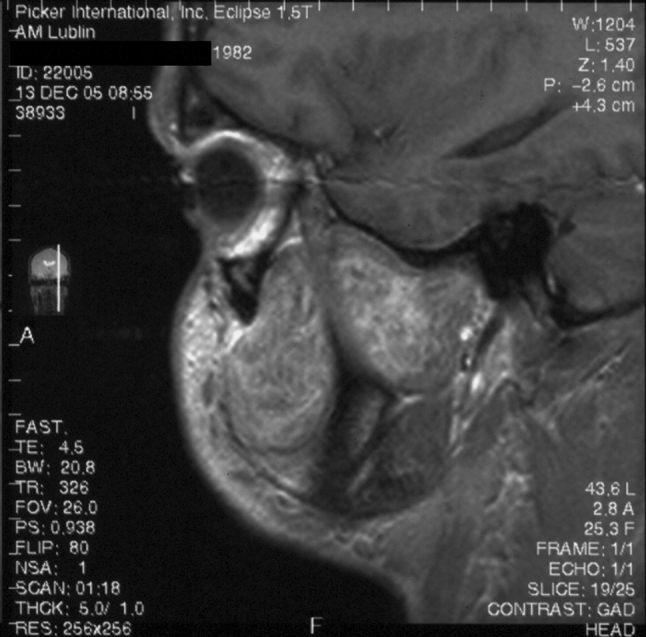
Magnetic resonance, saggital T1-weighted image after contrast administration. Large tumor in the infratemporal fossa and cheek shows intensive, inhomogenous contrast enhancement [25]
